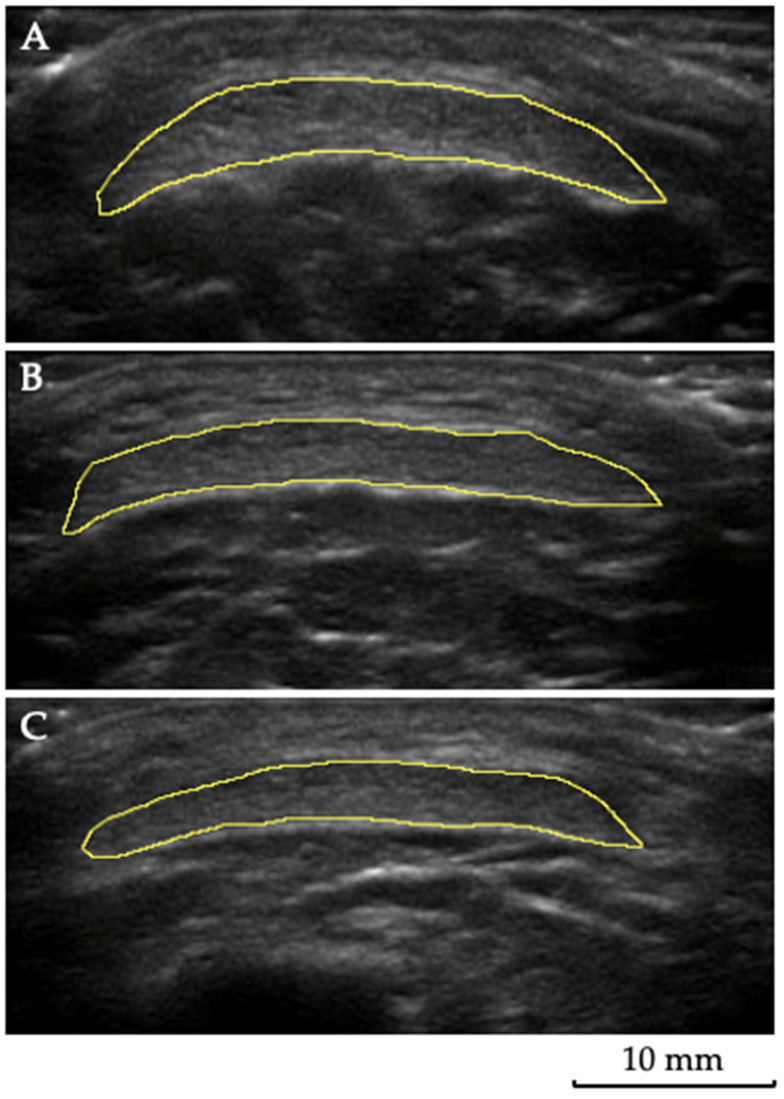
Tendon ultrasonography: a representative image of the patellar tendon ultrasound analysis. For this evaluation, the probe was placed at 25% (A), 50% (B), and 75%(C) of the length of the patellar tendon. The tendon contours were selected using the tracing technique to measure the cross-sectional area and echogenicity.
